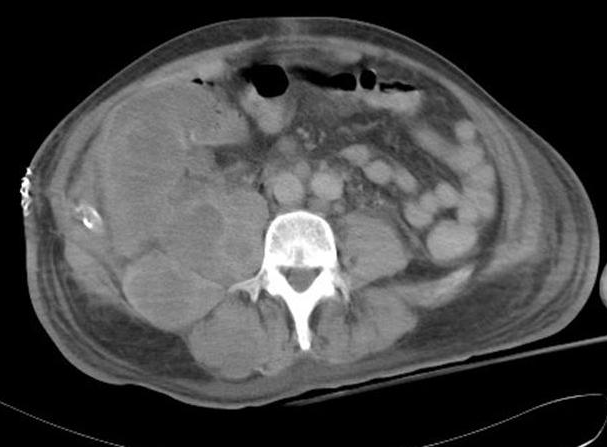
Computed Tomography image showing multiple recurrent lesions in the psoas muscle.
